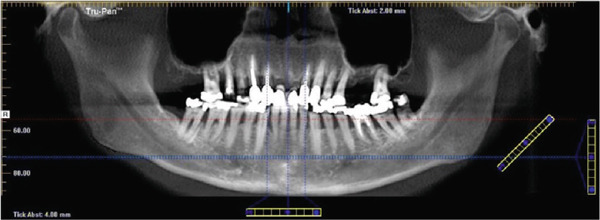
The panoramic radiograph was utilized to assess the present status of the dental pathologies and to document the surgical removal of the tonsil stones, as requested by the patient. A comparison with prior images reveals the absence of focal radiolucent structures in the region of the ascending branch of the mandibular ramus, thereby indicating the complete removal of the tonsil stones. The surgical site appears unremarkable, with no inflammation or other postoperative changes. The surrounding jaw structures remain intact, with no abnormalities detected. This image documents the successful procedure, confirming postoperative healing and the absence of recurrence.
